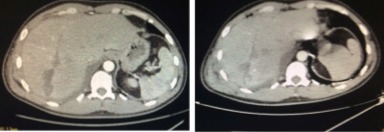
Abdominal CT at day 7 after ablation showing the cicatrization of the liver and resorption of initial perihepatic hemoperitoneum.
